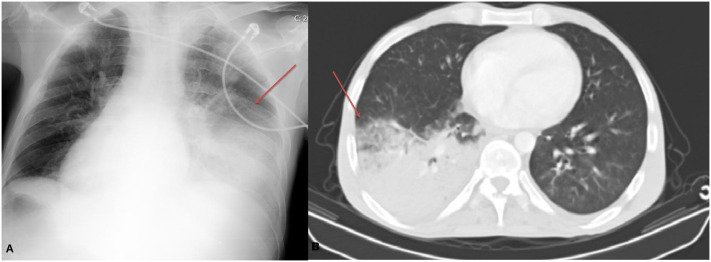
(A) Chest X-ray showing diffuse parenchymal opacities, more prominent in the right lower lung zone (arrow), consistent with consolidation. (B) Chest computed tomography (CT) image demonstrating dense consolidation with air bronchograms in the right lower lobe (arrow), compatible with pneumonia.
